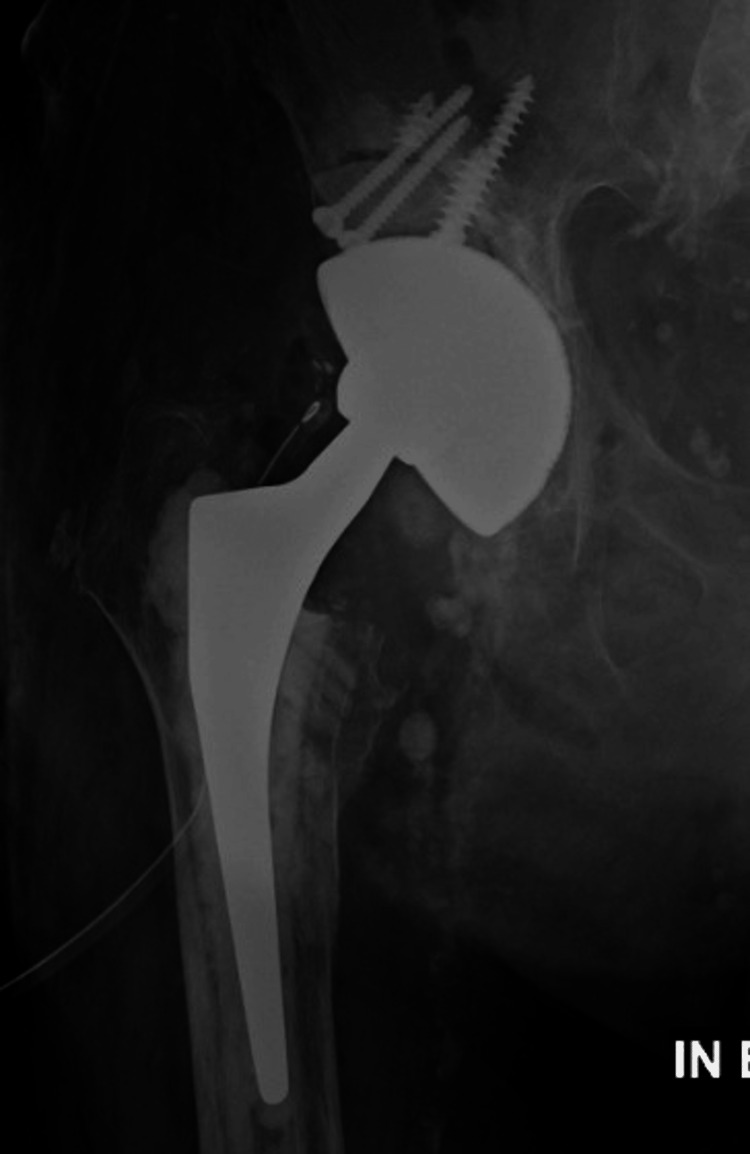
Postoperative X-ray three months after cup revision through the direct anterior approach.
